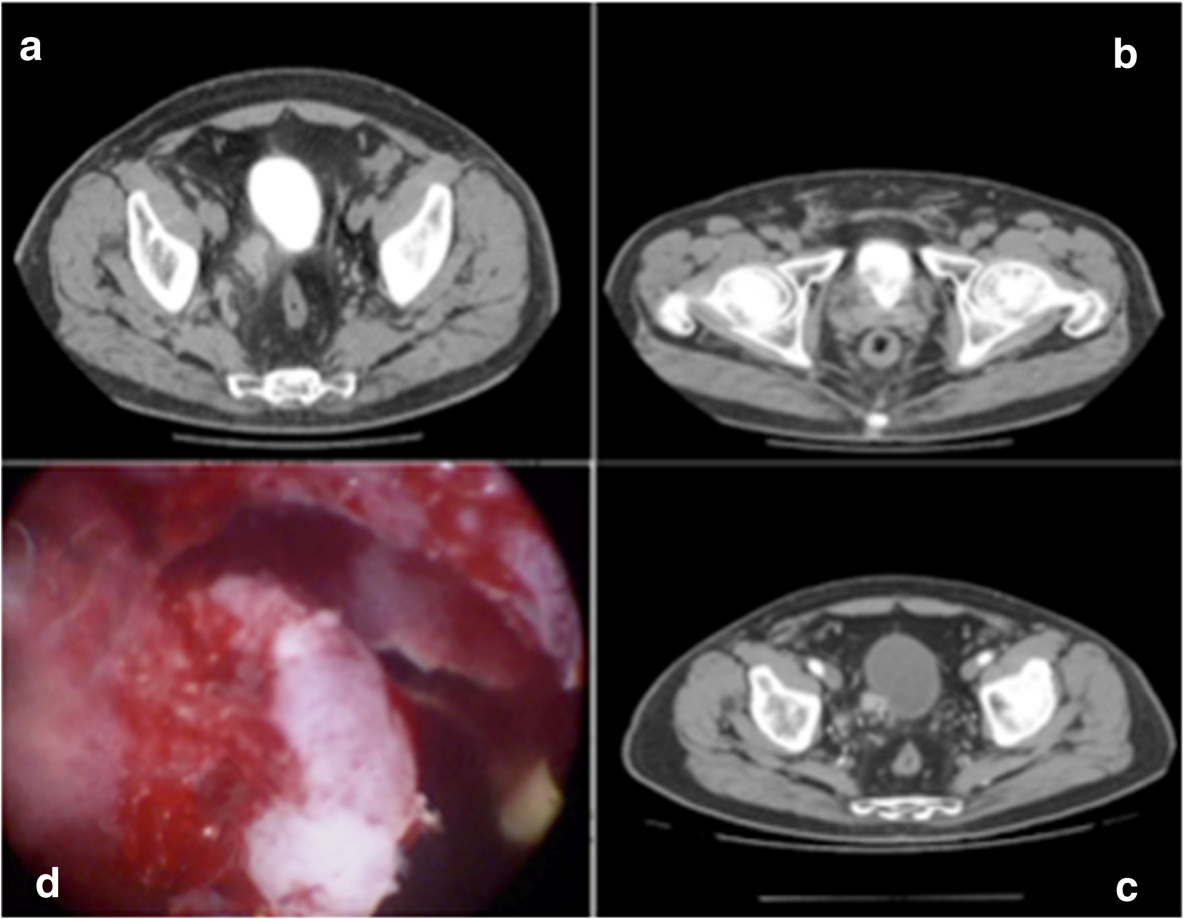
Computed tomography and transurethral resection of the bladder lesion. CT showed mild heterogeneous enhancement of the lesion, which infiltrated the bladder wall, no other lesions or local or systemic adenopathy were observed (a–c). Transurethral resection of bladder (d).
